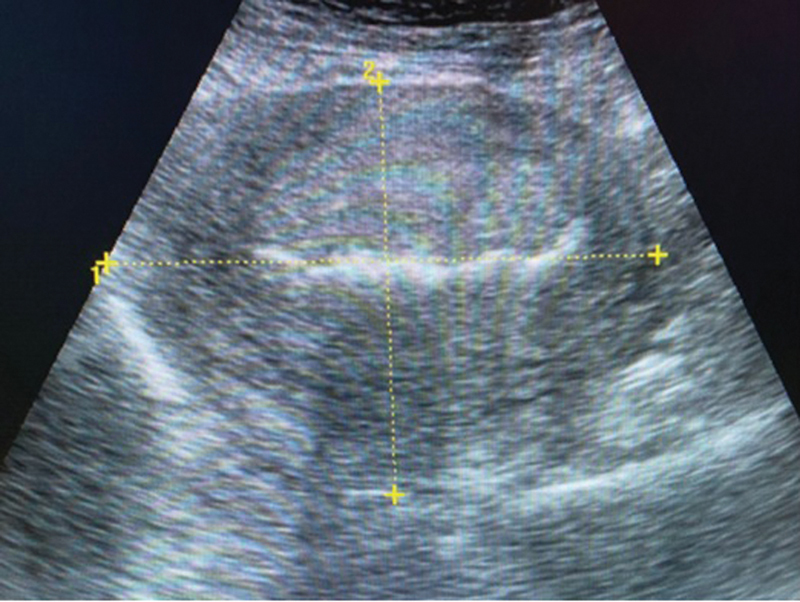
Immediate postpartum ultrasound: endometrial echogenic and uterus size evaluation.
